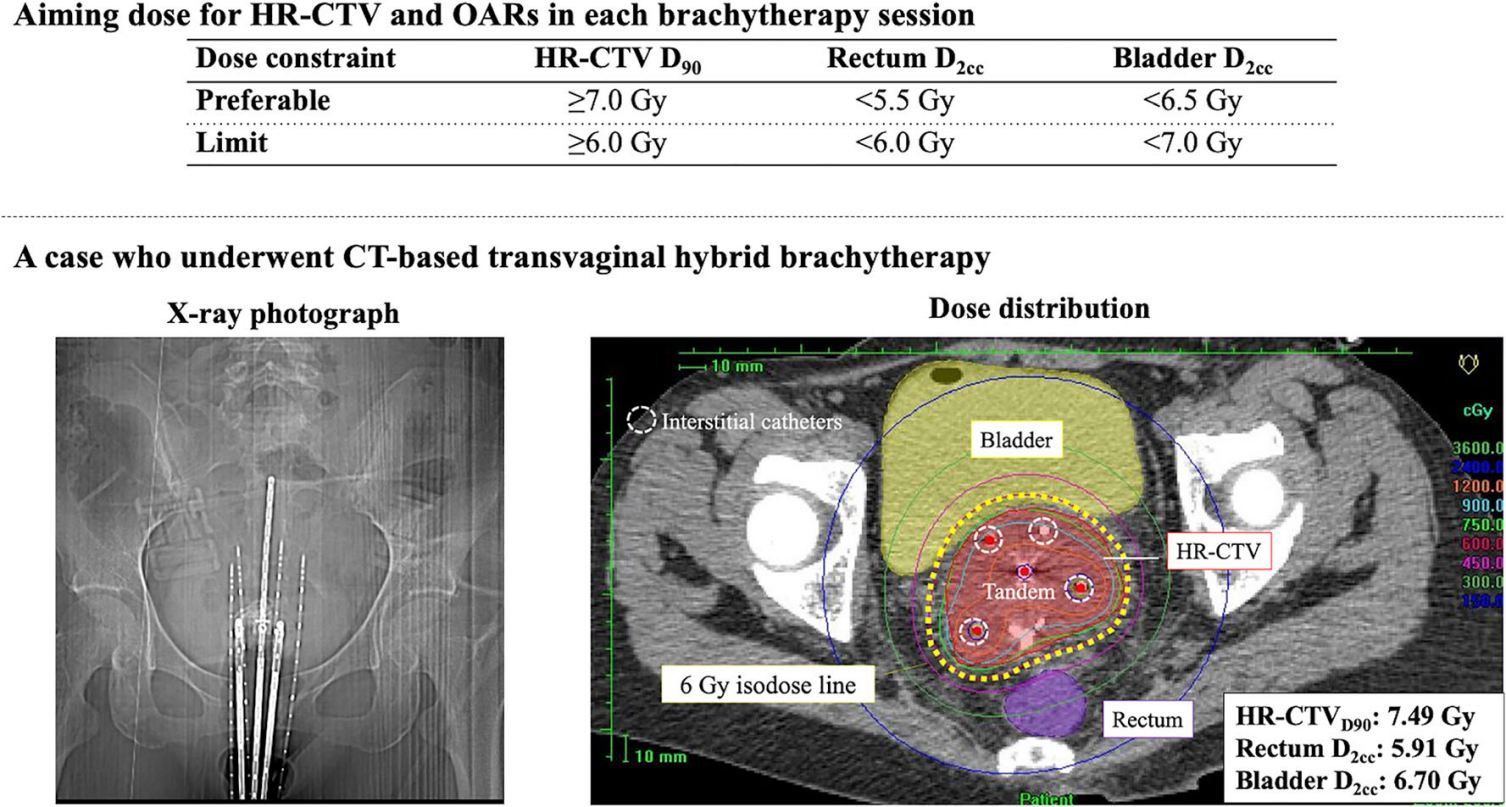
Aiming dose for HR‐CTV or OARs in each brachytherapy session and actual case who underwent CT‐based transvaginal hybrid brachytherapy. HR‐CTV, high‐risk clinical target volume; OARs, organs at risk
